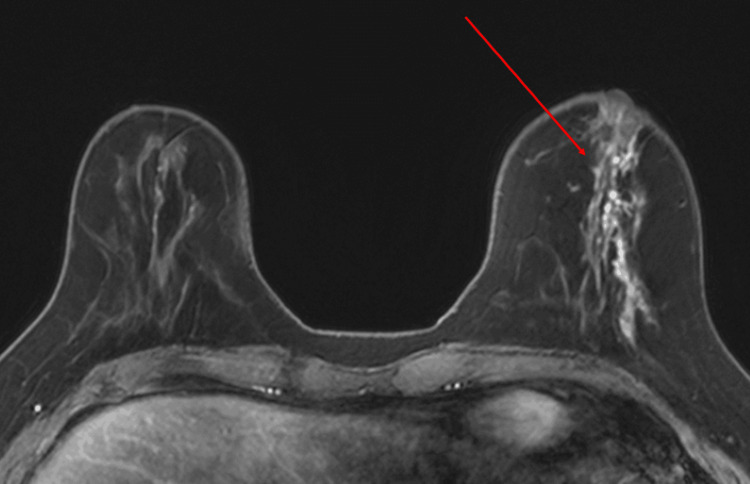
Breast MRI post-contrast dynamic: segmental non-mass enhancement with plateau kinetics in the central left breast (indicated by arrow).
